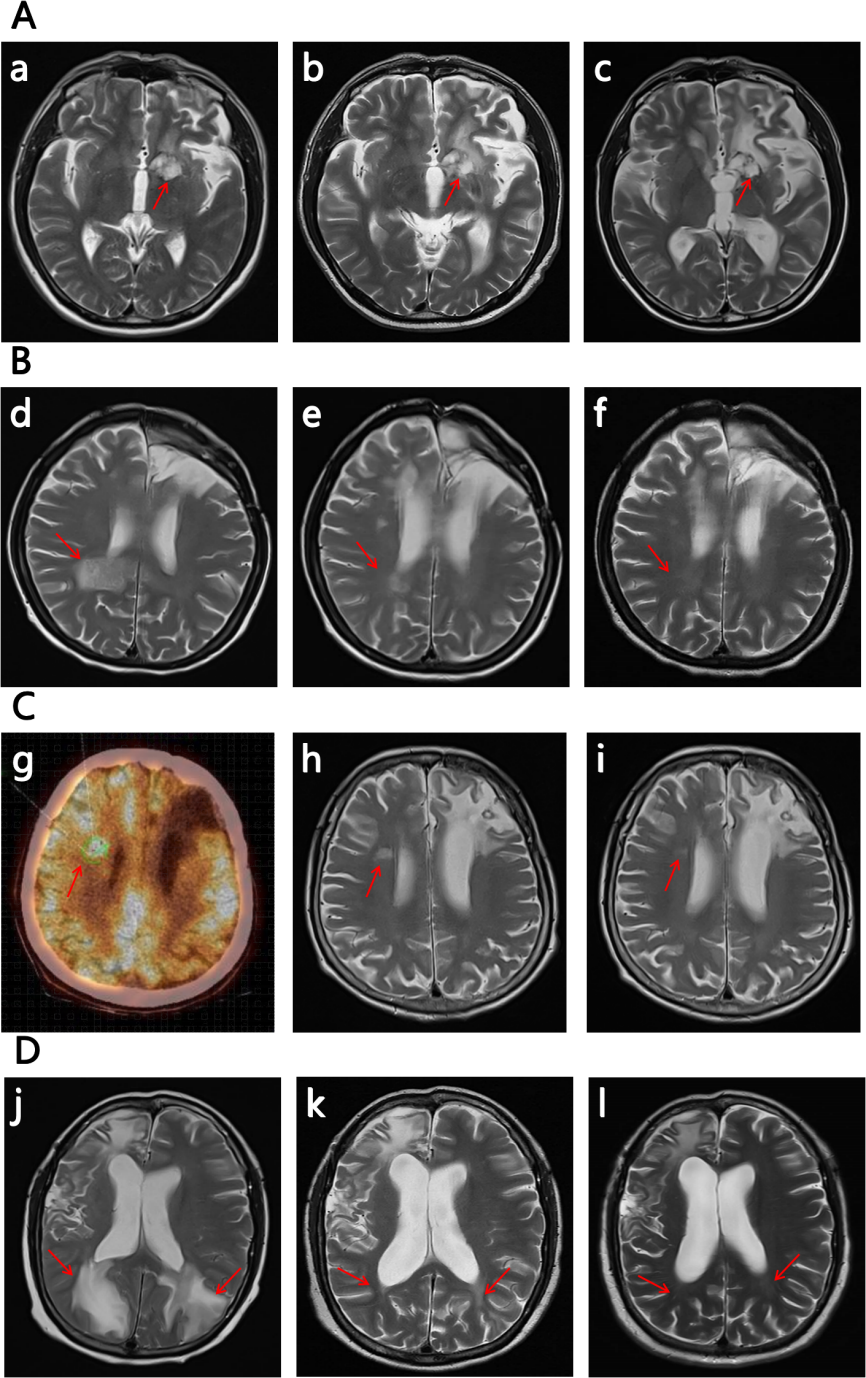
The images of the 4 R/R CNSL patient (the subfigure g was by PET-CT, while others were all by MRI). The significant decrease in the size and contrast enhancement of the CNS lesion (arrows) on T2-weighted post-gadolinium contrast axial images show the response to the treatment (for subfigure g the arrow indicates the location of relaspsed tumor). The positions pointed by the arrows are the locations of the tumor, and the other abnormalities on the images are due to the craniotomy. (A) No.1 patient: (a) on 2024/12/02 at relapse before the therapy of glofitamab combined with lenalidomide; (b) on 2025/02/15 after 2 cycles of the therapy; (c) on 2025/04/02 after 4 cycles of the therapy. (B) No.2 patient:(d) on 2024/10/19 at relapse before the therapy of glofitamab combined with lenalidomide; (e) on 2024/12/05 after 2 cycles of the therapy; (f) on 2025/01/25 after 4 cycles of the therapy. (C) No. 3 patient: (g) a PET-CT scan on 2024/12/02 at relapse before the therapy of glofitamab combined with lenalidomide; (h) on 2025/01/14 after 2 cycles of the therapy; (i) on 2025/03/07 after 4 cycles of the therapy. (D) No. 4 patient. (j) on 2025/03/28 at relapse before the therapy of glofitamab combined with lenalidomide; (k) on 2025/05/15 after 2 cycles of the therapy; (l) on 2025/06/23 after 4 cycles of the therapy.
